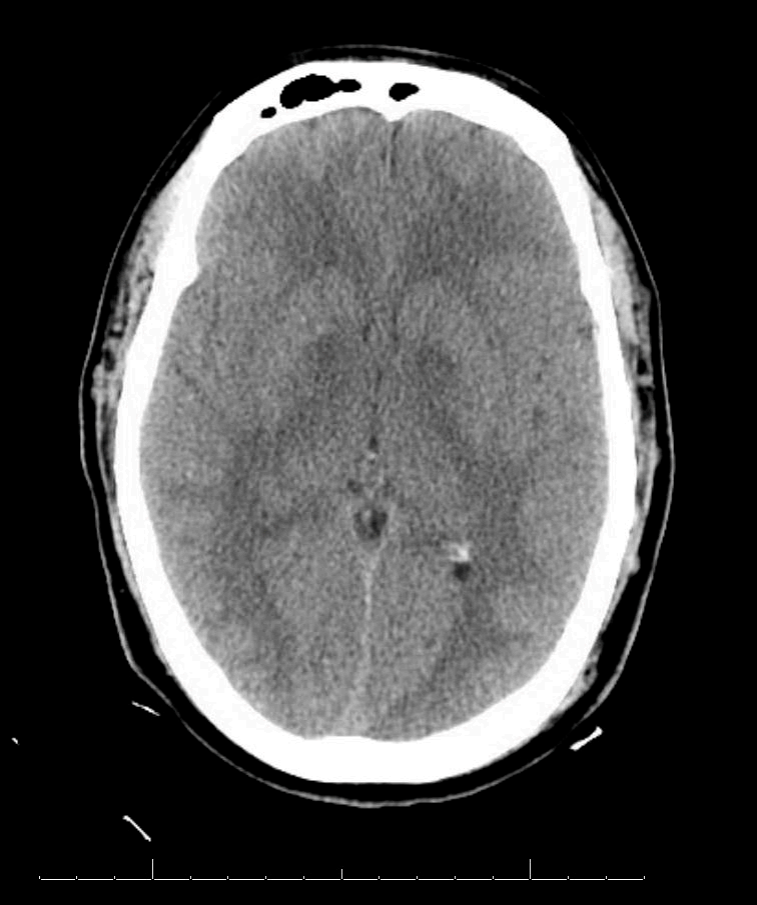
CT Head Without Contrast Demonstrating Symmetric Hypodensity of Bilateral Globus Pallidus (GP)
